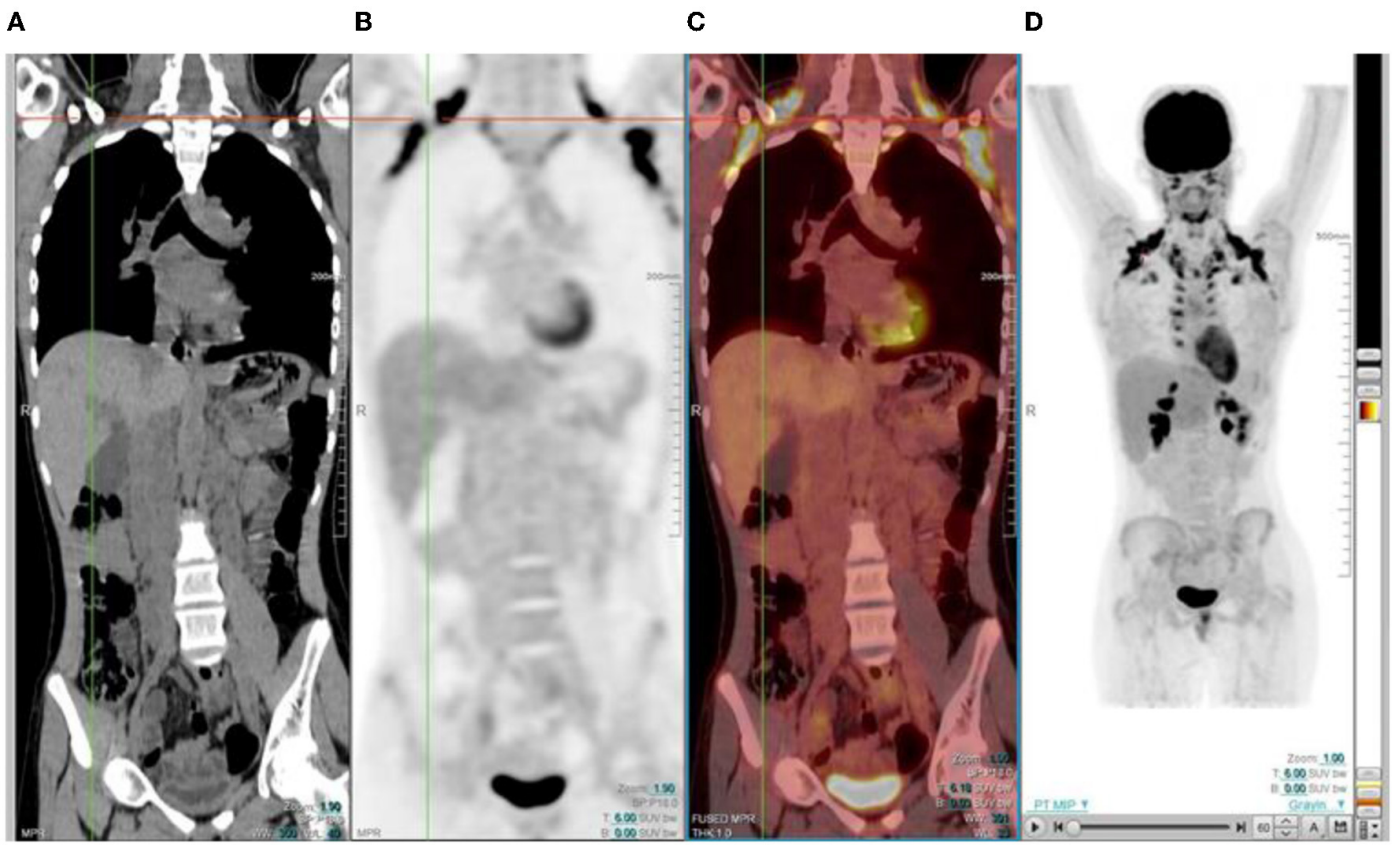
15 year old male with stage 3 Hodgkin's lymphoma referred for follow up FDG PET post chemotherapy. Coronal PET (B), and PET/CT (C) showing bilateral symmetrical uptake in the neck and paravertebral region of the cervical and thoracic spine. Crosshairs localized to fat density on CT (A). FDG uptake in brown adipose tissue is commonly seen in children and adolescents. Many pediatric patients have mild brown fat uptake in the neck or supraclavicular regions. However, intense uptake may include pericardiac and perirenal brown adipose tissue. ^18^F-FDG uptake in activated brown fat may obscure sites of pathologic FDG uptake and decrease confidence in the interpretation of the study.
